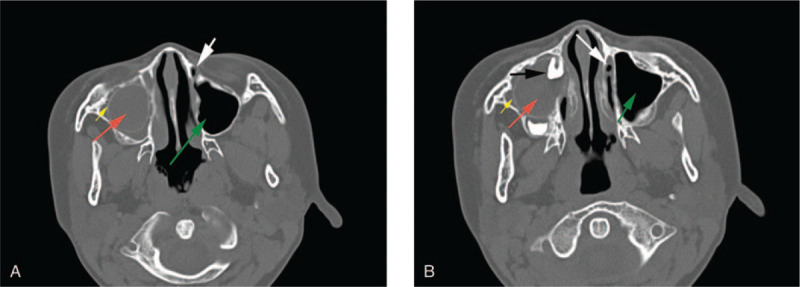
CT imaging of the ectopic tooth, the dentigerous cyst and the blocked nasolacrimal canal. Red arrow indicates the low-density image wrapped by a high density loop (yellow arrow) in the right maxillary sinus; green arrow indicates the left normal maxillary sinus. White arrow indicates the left normal nasolacrimal canal, while at the corresponding right position, the nasolacrimal canal is disappeared. Black arrow indicates a tooth in the cyst.
